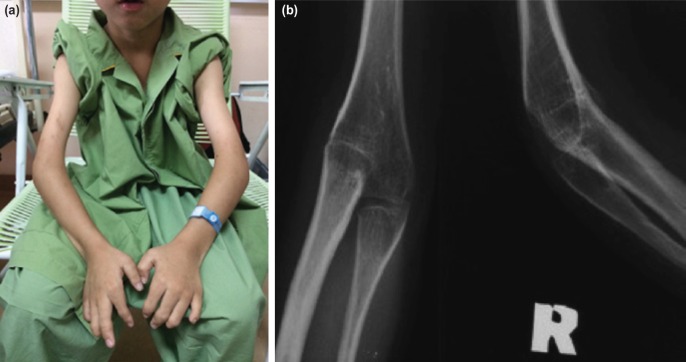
(a) Pre-operative photograph of the patient’s bilateral elbows in fixed flexion of 30 degrees. (b) The pre-operative right elbow radiograph demonstrating humeroradial synostosis with hypoplastic humeroulnar joint.
